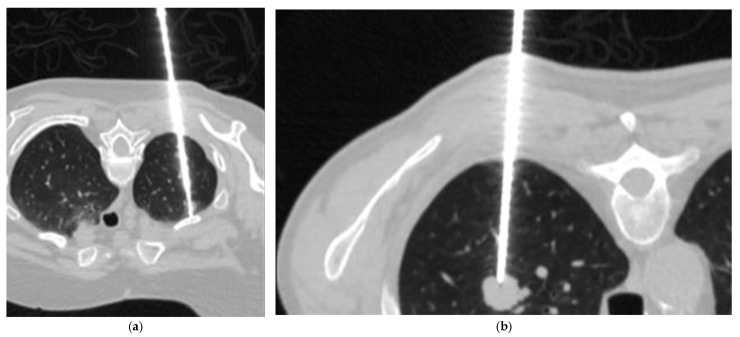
CT-guided transthoracic lung biopsy. (a) CT-TTB was performed with the patient in the prone position. The coaxial needle is advanced toward the target lesion, and an 18-gauge semi-automated biopsy needle is deployed through the coaxial system, as visualized on a low-dose control CT scan. (b) CT-TTB showing the coaxial needle positioned adjacent to the target lesion prior to tissue sampling. Following stylet removal, a biopsy was performed using an 18-gauge automated core biopsy needle.
